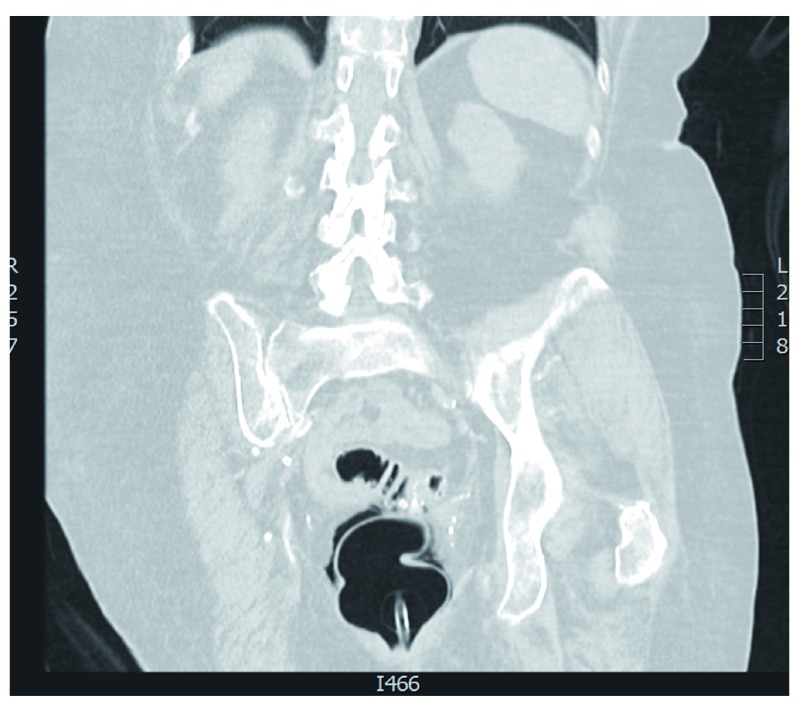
CT colonography coronal view depicting rectal perforation. The Foley catheter is seen within the rectum as well as peri-rectal air.
